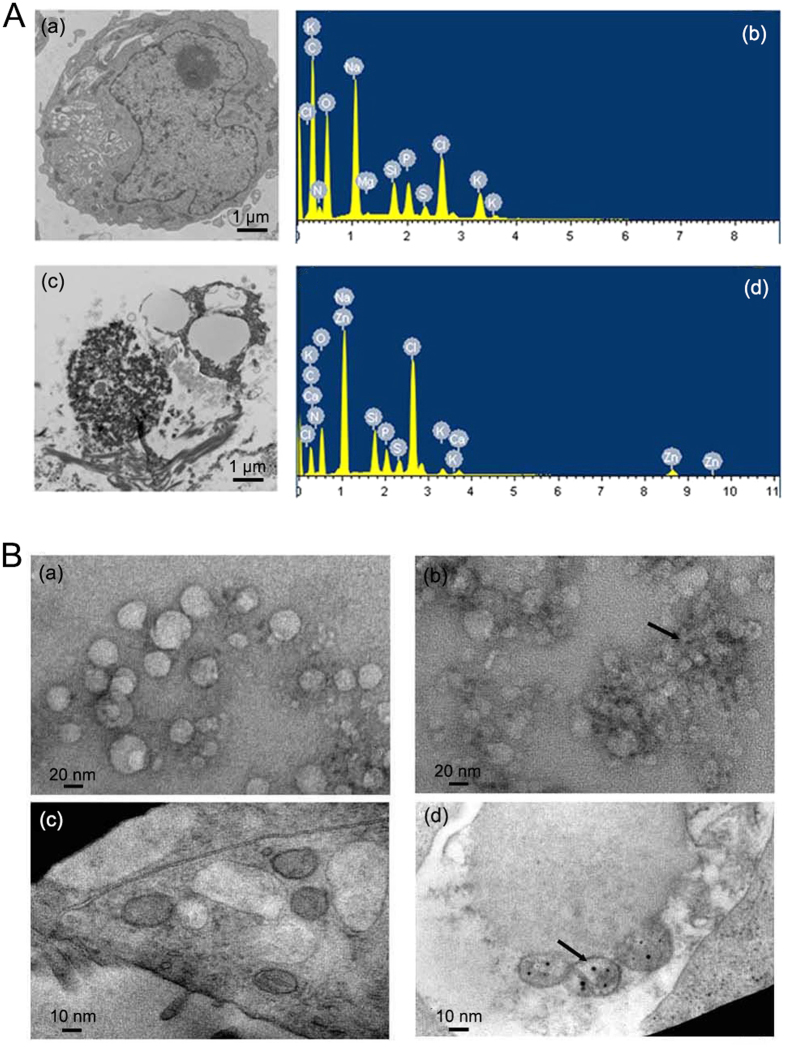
(A) Morphological and compositional characterization of Zn^2+^-treated tumor cells: (a) and (c) show TEM images at the sub-cellular level of control and Zn^2+^-treated cells, respectively; (b) and (d) show the corresponding results of the EDS analysis. (B) TEM images of MVs from (a) Zn^2+^-treated KA cells; (b) Zn^2+^-treated KA cells under ultrasound treatment ( → ); (c) KA cells without treatment; (d) Zn^2+^-treated KA cells under ultrasound treatment (→).
